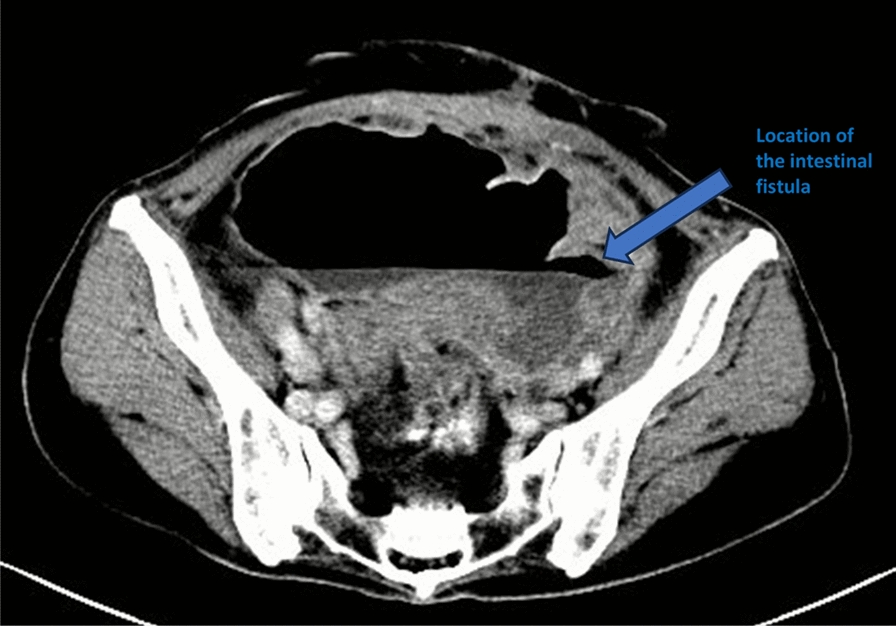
Postoperative computed tomography image demonstrates a localized fluid collection and extraluminal air secondary to an enteric fistula, with the arrow indicating the site of the fistula
